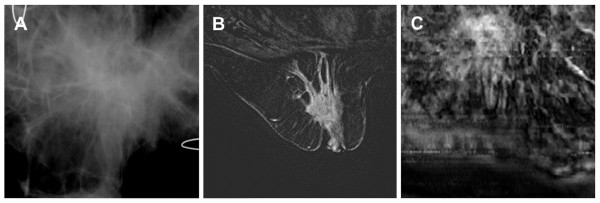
Images from case 4. (A) Mammogram shows a large distorted area with spiculation. The U-shaped wires are used to confirm image orientation. (B) Magnetic resonance image shows a large, irregular, and spiculated region. The main mass measured approximately 8.5 × 3.3 × 5.4 cm. (C) Vibro-acoustography image at a 2-cm depth shows a large spiculated mass. Note that figure parts (A) and (C) show only a 5 × 5-cm area within the breast, whereas part (B) shows the entire breast.
